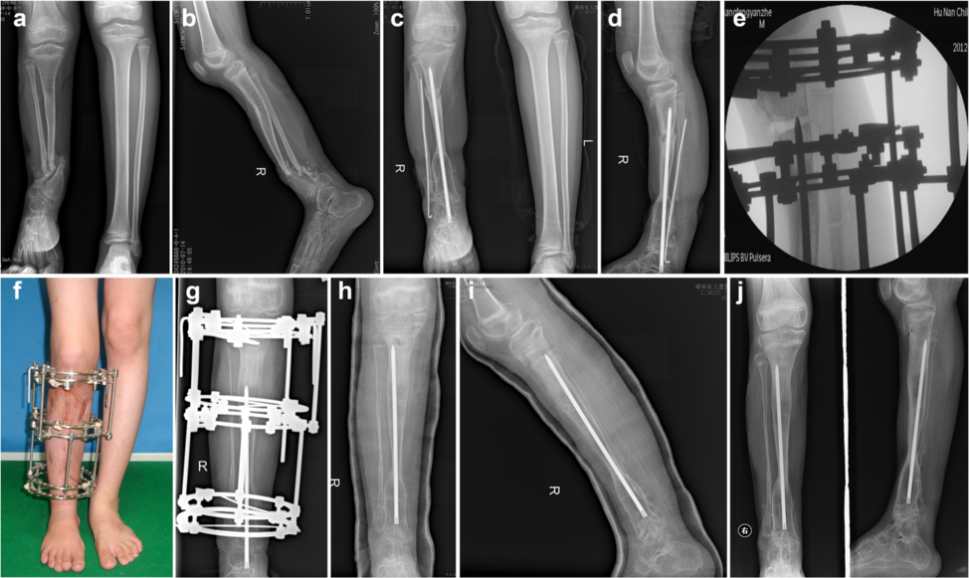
Another representative case (Patient 6). A 14.5y boy with CPT associated with neurofibromatosis type 1 (NF1) had radiological findings of proximal tibial dysplasia (a, b). Then a combined Ilizarov fixator with intramedullary rodding of the tibia and wrapping autogenic iliac bone graft technique was carried out to manage the pseudarthrosis. And simultaneously a proximal tibial osteotomy was implemented to have access to the normal alignment with the same intramedullary rodding. Two years after the procedure, the radiography showed a primary union of pseudarthrosis but nonunion of osteotomy site with LLD of 8 cm(c, d). A proximal tibial lengthening and compression of the primary osteotomy site was carried out to equalize the limb length (e– g). The length gained was 8 cm with HI of 68 d/cm. Then the ex-fixator was removed and the intramedullary rod was pushed into the tibia cavity. The radiography showed both the lengthening segment and the primary osteotomy site were union (h, i). One year after removal of ex-fixator, X rays showed well corticalization of the lengthening segment with good alignment of tibia, however the ankle joint was stiff with degenerative changes (j)
